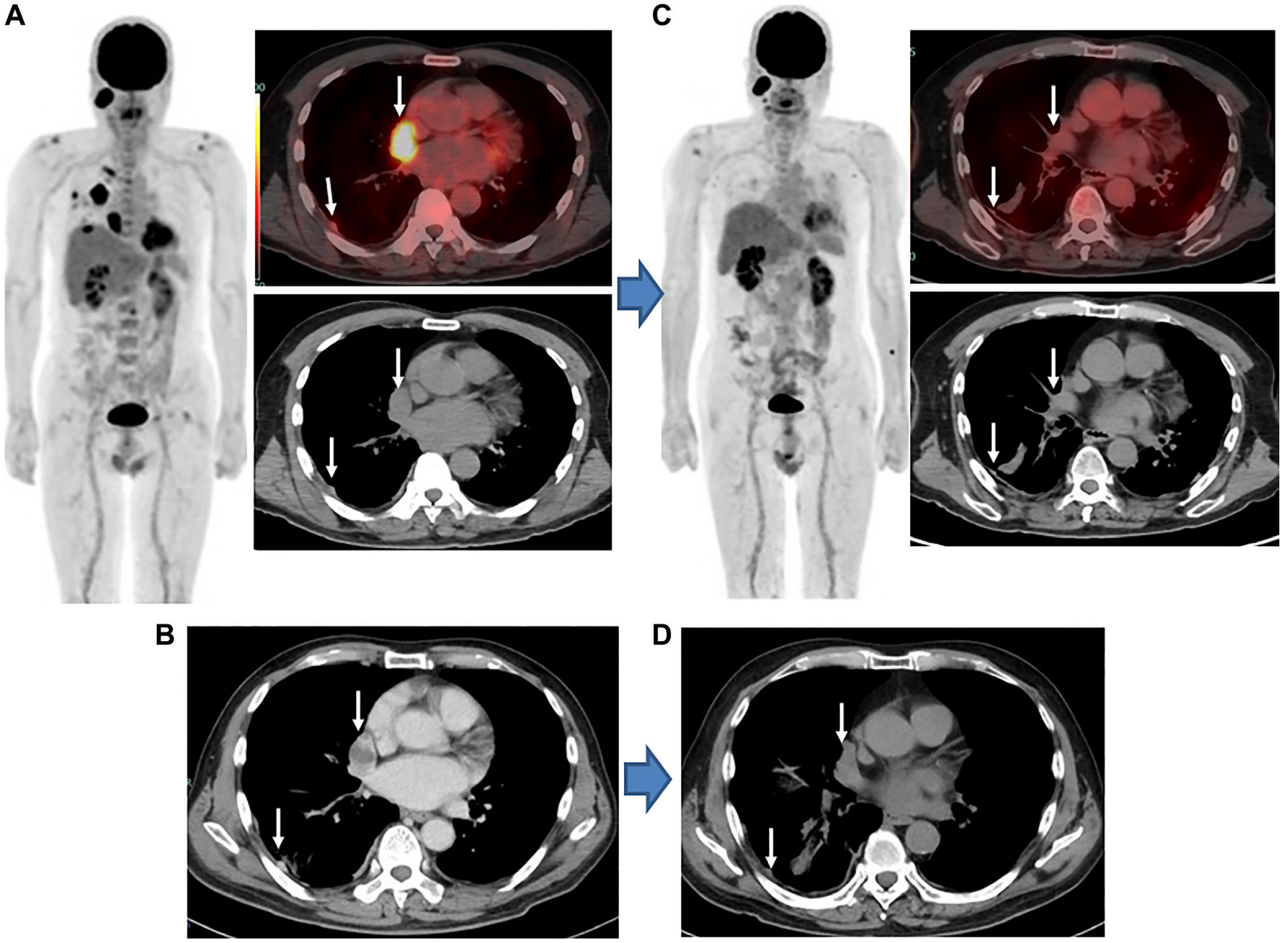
Sarcomatoid MPM, stage IB (cT3N0M0), man, 70 years old. (A) Findings obtained with FDG-PET/CT prior to combined nivolumab and ipilimumab immunotherapy indicated several areas in right pleural lesions with high levels of FDG uptake (arrows). Tumor-like FDG uptake in parotid gland on the right side indicates a Warthin tumor. (B) Findings obtained with contrast-enhanced CT findings prior to combined nivolumab and ipilimumab immunotherapy indicated a mass-forming thickness in the right pleura (arrows). (C) Following four cycles of combined nivolumab and ipilimumab treatment, FDG-PET/CT findings showed FDG uptake disappearance (lower than mean liver activity) in pleural lesions (arrows). (D) Following four cycles of combined nivolumab and ipilimumab, non-contrast CT findings showed mild improvement in pleural lesions (arrows). imPERCIST findings showed CMR, while mRECIST indicated PR classification, with a decrease in total size of six pleural lesions perpendicular to the chest wall of 47.1%. At 12.1 months following initiation of combined immunotherapy, progression was noted and the patient died at 16.8 months.
